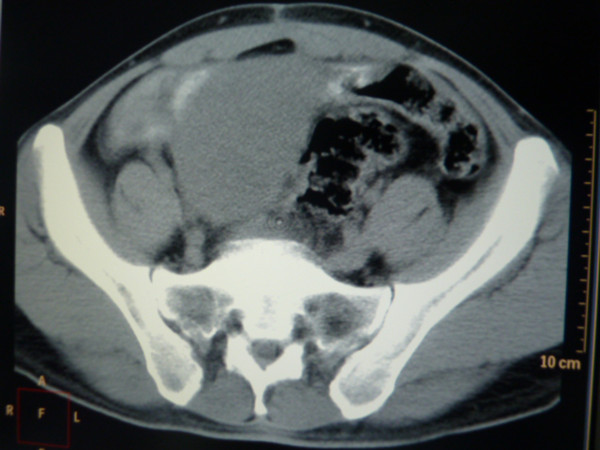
CT Imaging after three cycles of neoadjuvant chemotherapy. CT, computed tomography.
